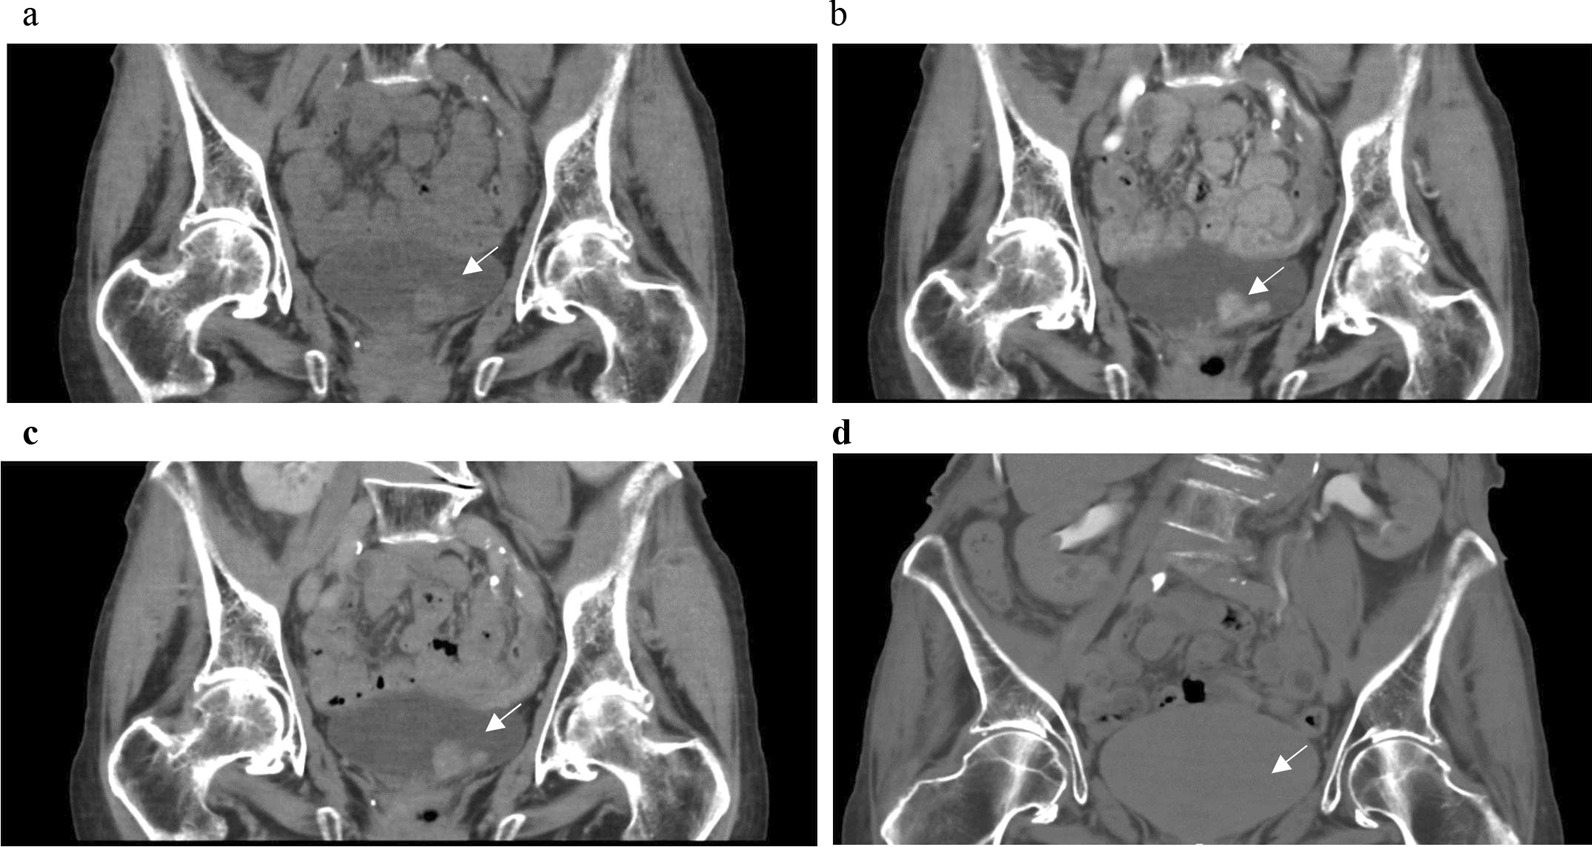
Urinary bladder cancer attenuation in a coronal computed tomography urography reconstruction in: a unenhanced phase, b corticomedullary phase (CMP), c nephrographic phase, and d excretory phase. Notice that the tumor is clearly seen in CMP due to good enhancement and it is hidden in the not optimal contrast-mixed urine in the bladder in (d), sometimes happening despite efforts to get high concentration and volume of contrast in the bladder
